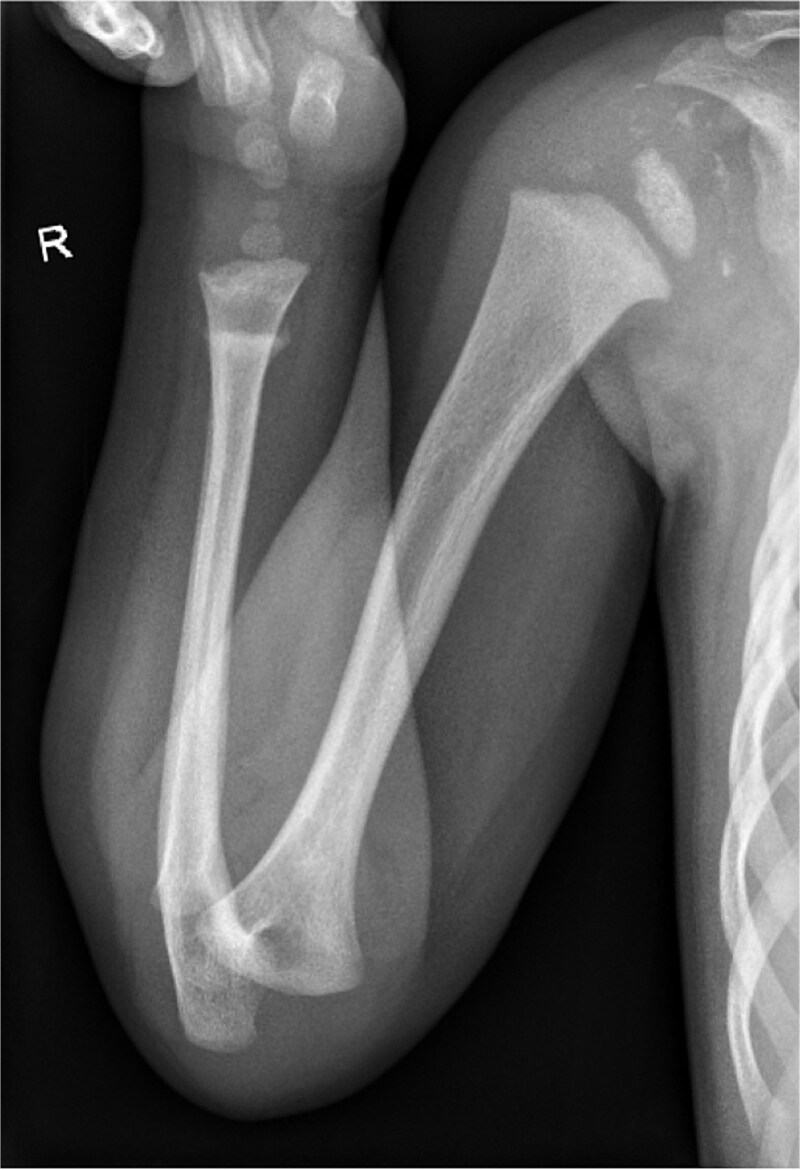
Radiographs demonstrating extensive right glenohumeral peri-articular calcification.
